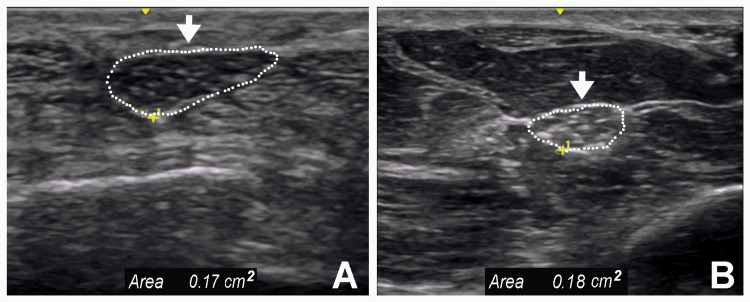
Ultrasound Study of the Right Median Nerve Short axis view of the right median nerve (arrow) showing a uniform increase in its cross-sectional area (A) at the distal wrist crease (17 mm^2^; normal: < 12 mm^2^) and (B) the mid forearm (18 mm^2^; normal < 8 mm^2^)
